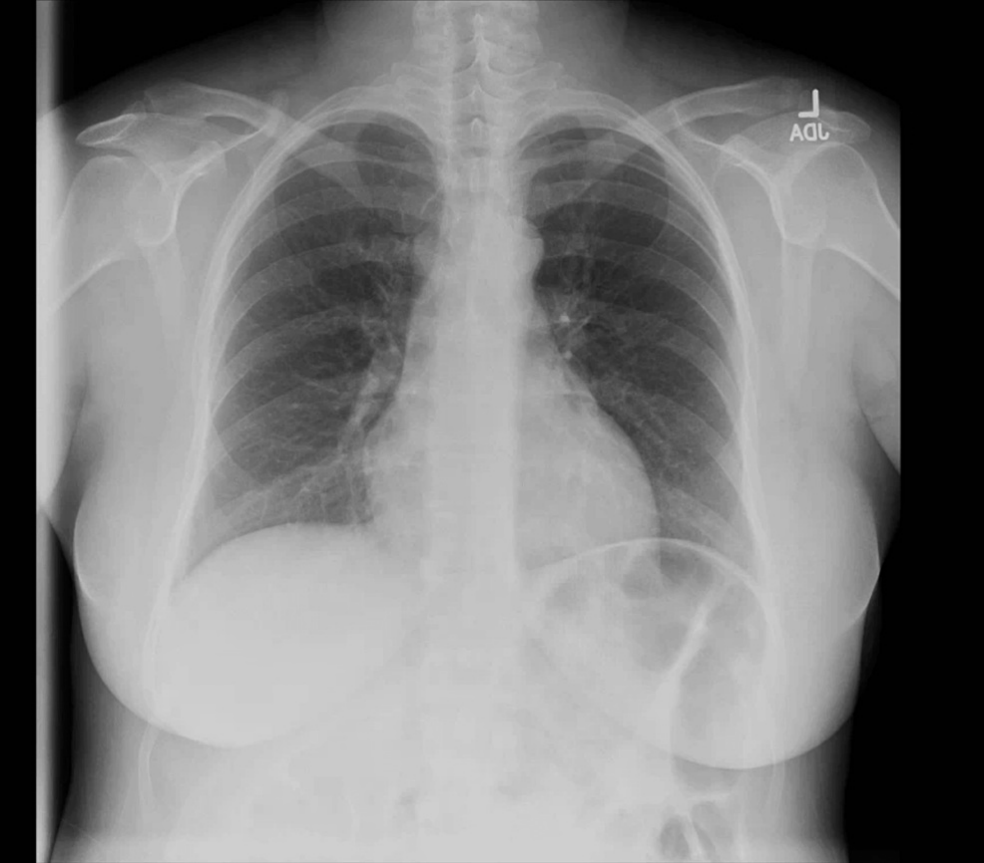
X-ray of the chest with no significant findings
